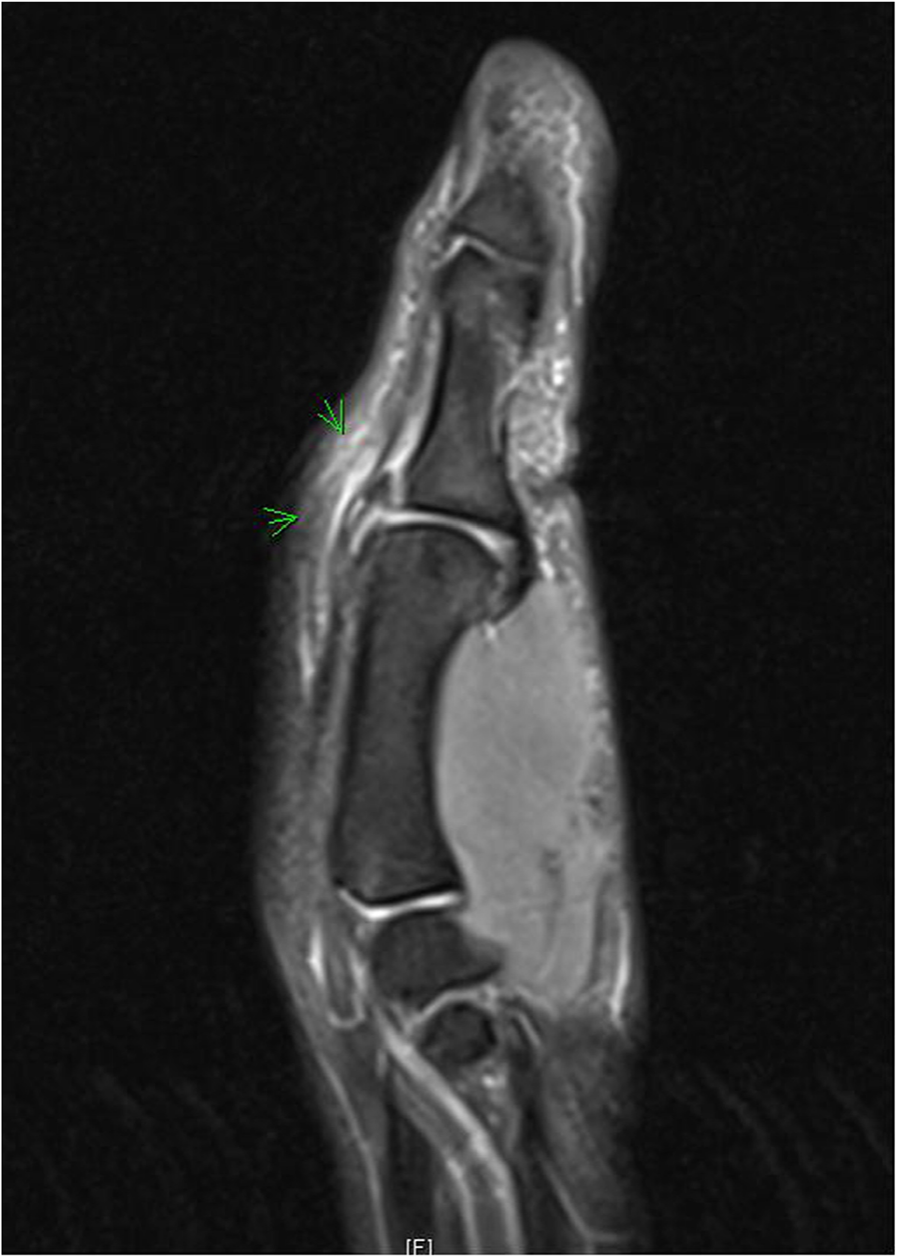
MRI of an old avulsion fracture of the UCL insertion.
